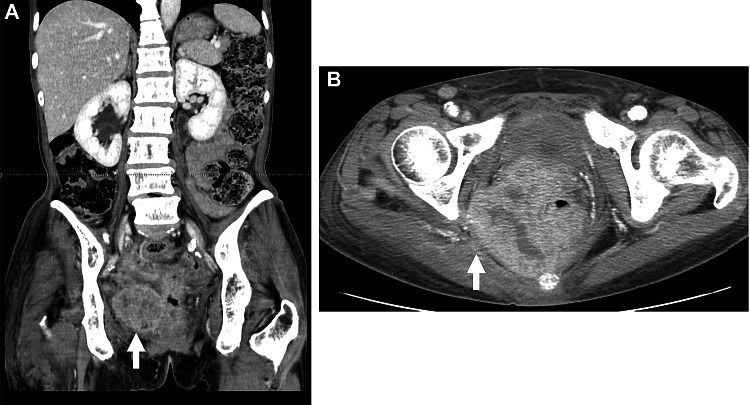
Abdomino-pelvic CT scan on admission. The tumor originating from the rectum had grown into the extra-rectal wall (white arrow: a) and was suspected of having invaded the gluteus maximus (white arrow: b)
